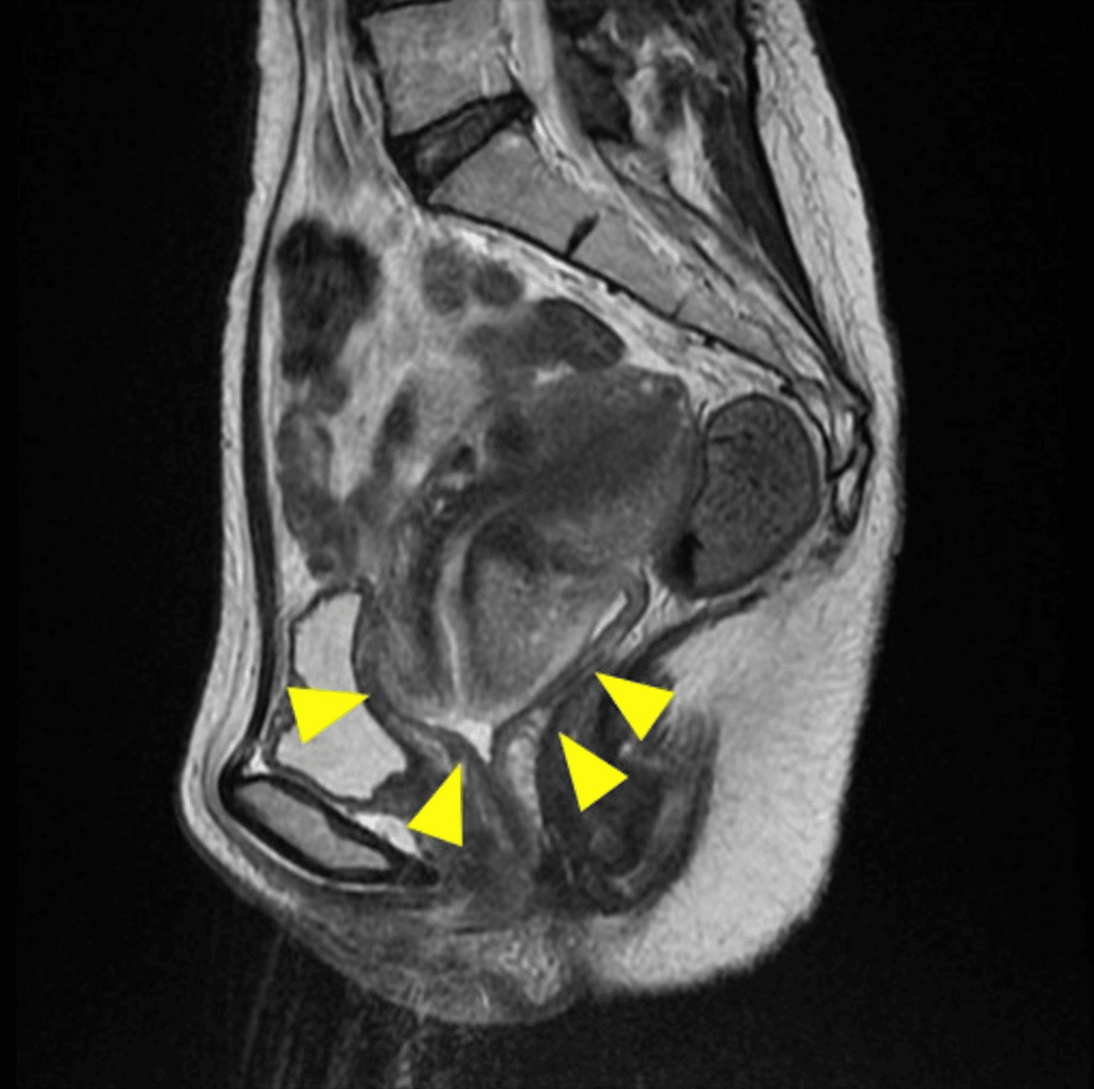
Magnetic resonance imaging (MRI) findings. MRI showing edematous cervical swelling with a preserved junctional zone (yellow arrowheads), without any findings suggestive of malignancy.
